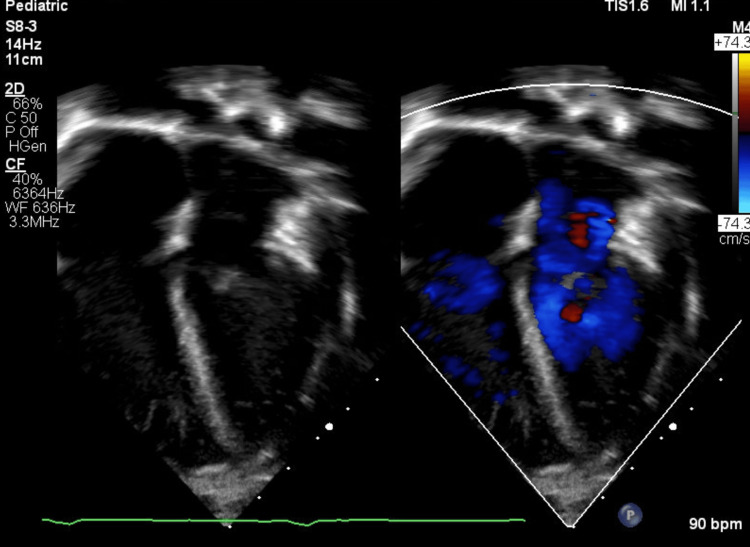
Post-operative four chamber view echocardiogram Post-operative echocardiogram showing a normal right atrium with no mass, and a normal tricuspid valve.
